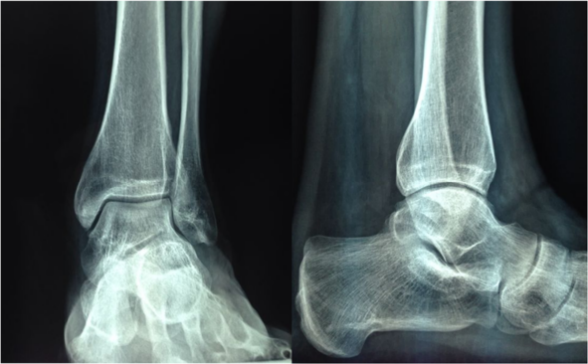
Anteroposterior and lateral radiographs of the left ankle in a soccer player showing signs of moderate osteoarthritis of the ankle
